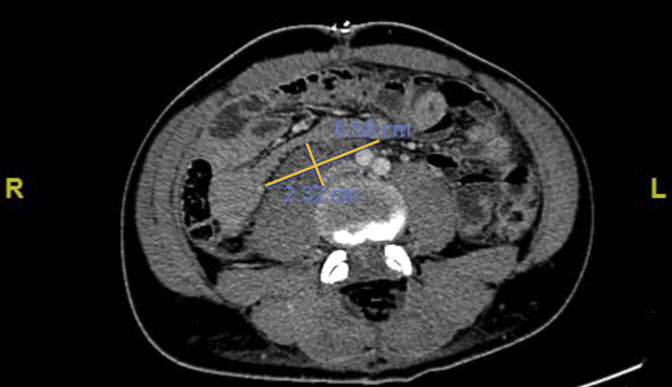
Axial CT cuts showing a smaller (2.3 × 6.6 cm) retroperitoneal hematoma.
